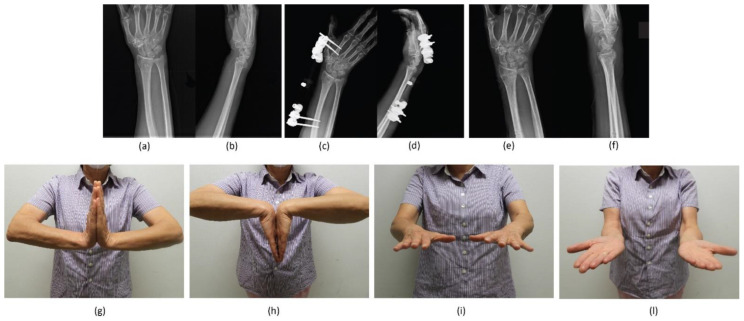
A 69-year-old female patient treated with BEF for an AO 23-B3 DRF. Antero-posterior (AP) and latero-lateral (LL) radiographic images at (a,b) pre-operative period; (c,d) immediate post-operative period; (e,f) at 2-month follow-up. Clinical-functional images showing: (g) extension, (h) flexion, (i) pronation, and (l) supination of the operated wrist at last follow-up.
